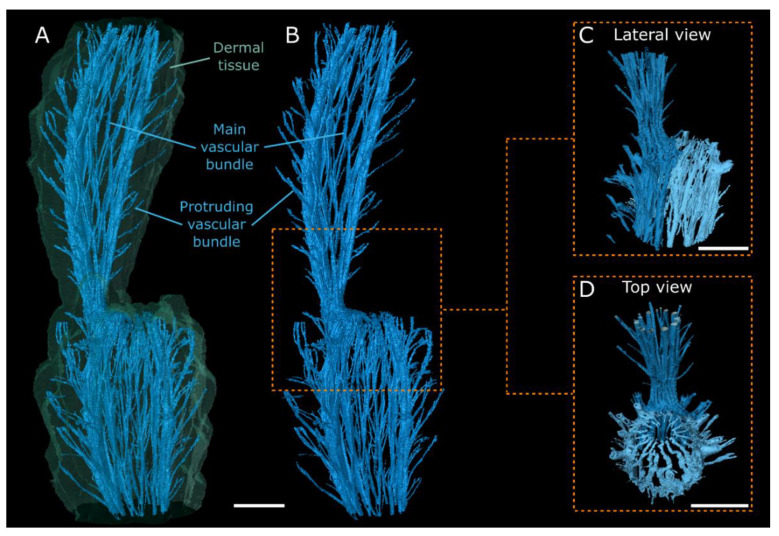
MRI scans of *Cylindropuntia bigelovii*. 3D representation of the dermal tissue (A); transparent green) and the vascular system ((A–D); coloured blue) of the junctions and parts of the connected lateral and sub-lateral branches, based on the segmentation of MRI scans. (A,B) Visualisation (lateral view) of an overview scan (sample 1). (C,D) Visualisation of a surface coil scan (sample 2) with a distinction between vascular structures that supply the lateral branch (dark blue) and those that run in a dormant bud (light blue). All scale bars equal 1 cm.
